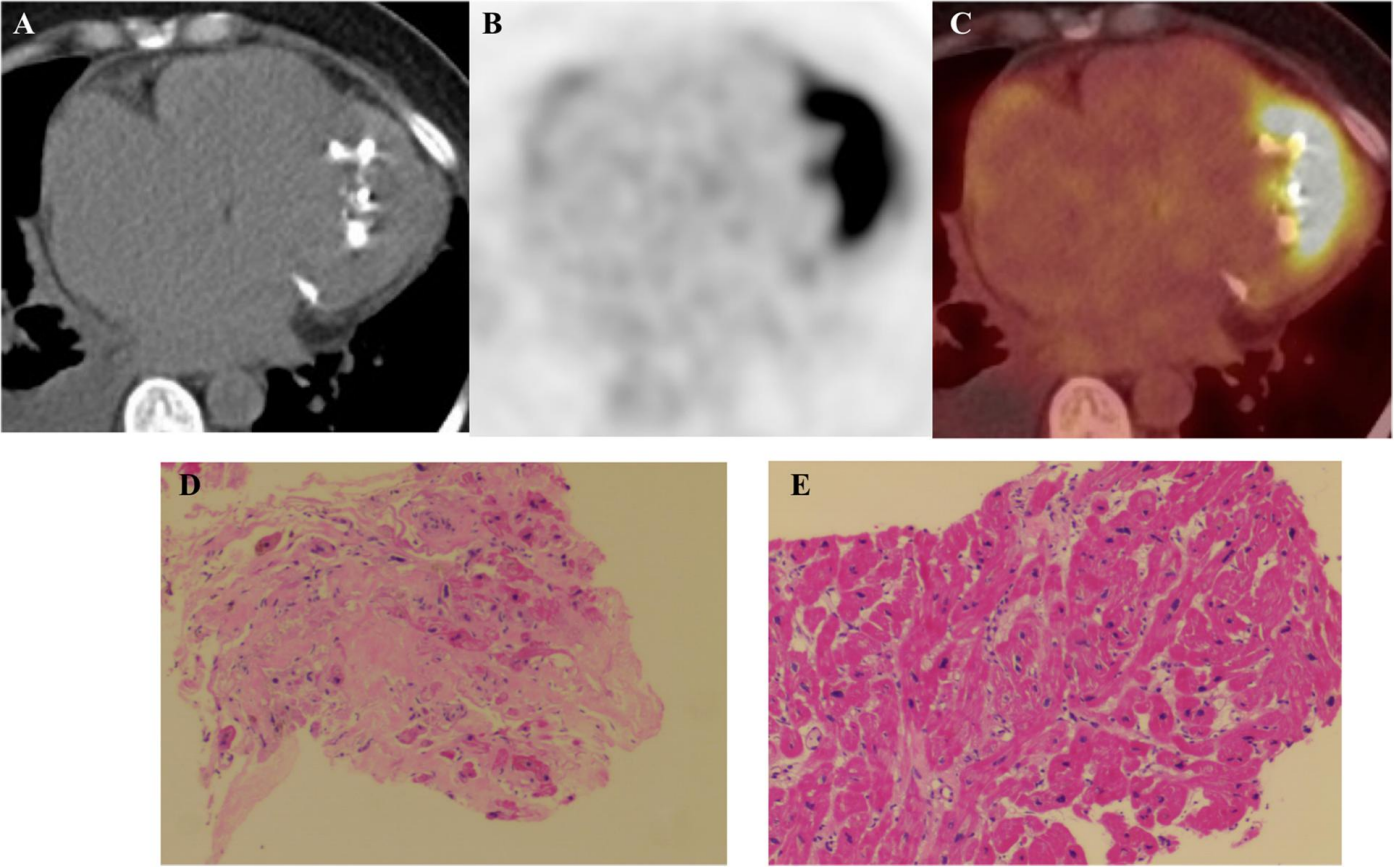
(A) Cardiac computed tomography shows marked calcification in the apex of the left ventricle (red arrow). (B,C)
